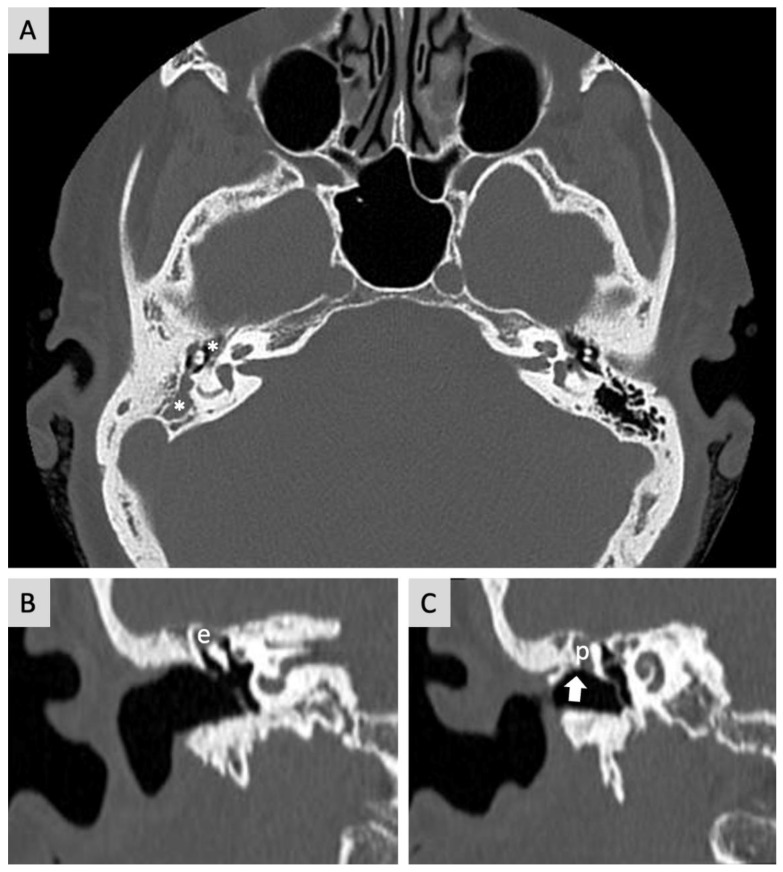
Head CT. (A) Axial—opacification of mastoid cells and middle ear on the right side (*). (B,C) Coronal—filling of the epitympanum (e) and Proussak space (p) with erosion of the spur of Chaussé (arrow) in the right ear.
